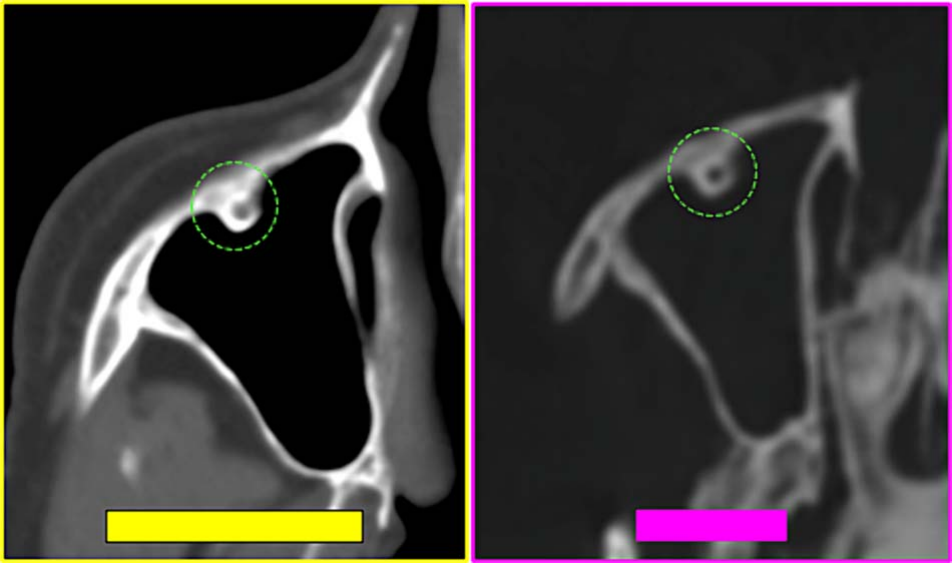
Coinciding morphology details of bone relief on the anterior wall of the right maxillary sinus (circumference) in the antemortem (left) and postmortem (right) CT scans.
